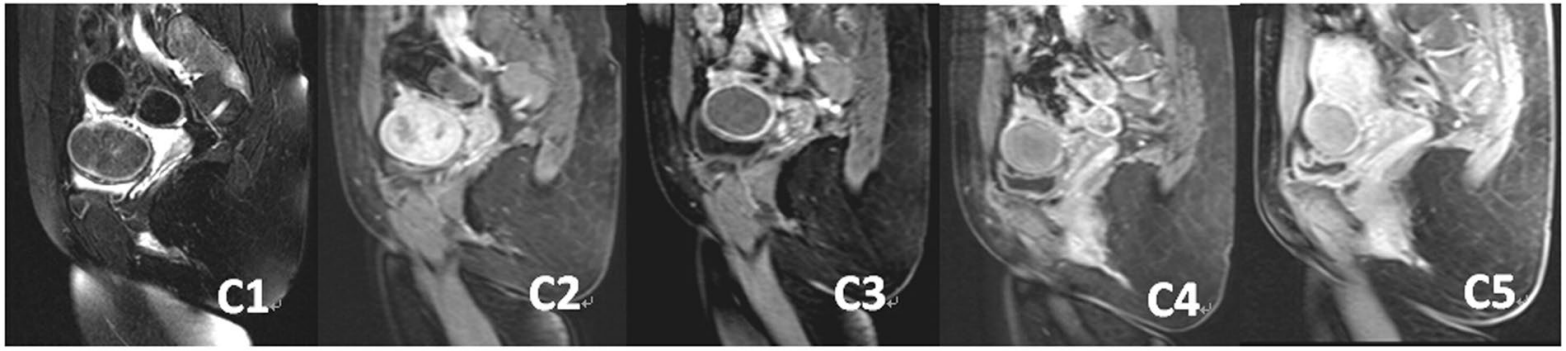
MRI of hypointense fibroid before and after HIFU treatment:C1, T2WI-MRI before treatment; C2, fibroid shows enhancement on contrast-enhanced MRI before treatment; C3, 3 months after treatment; C4, 6 months after treatment; C5, 12 months after treatment.
